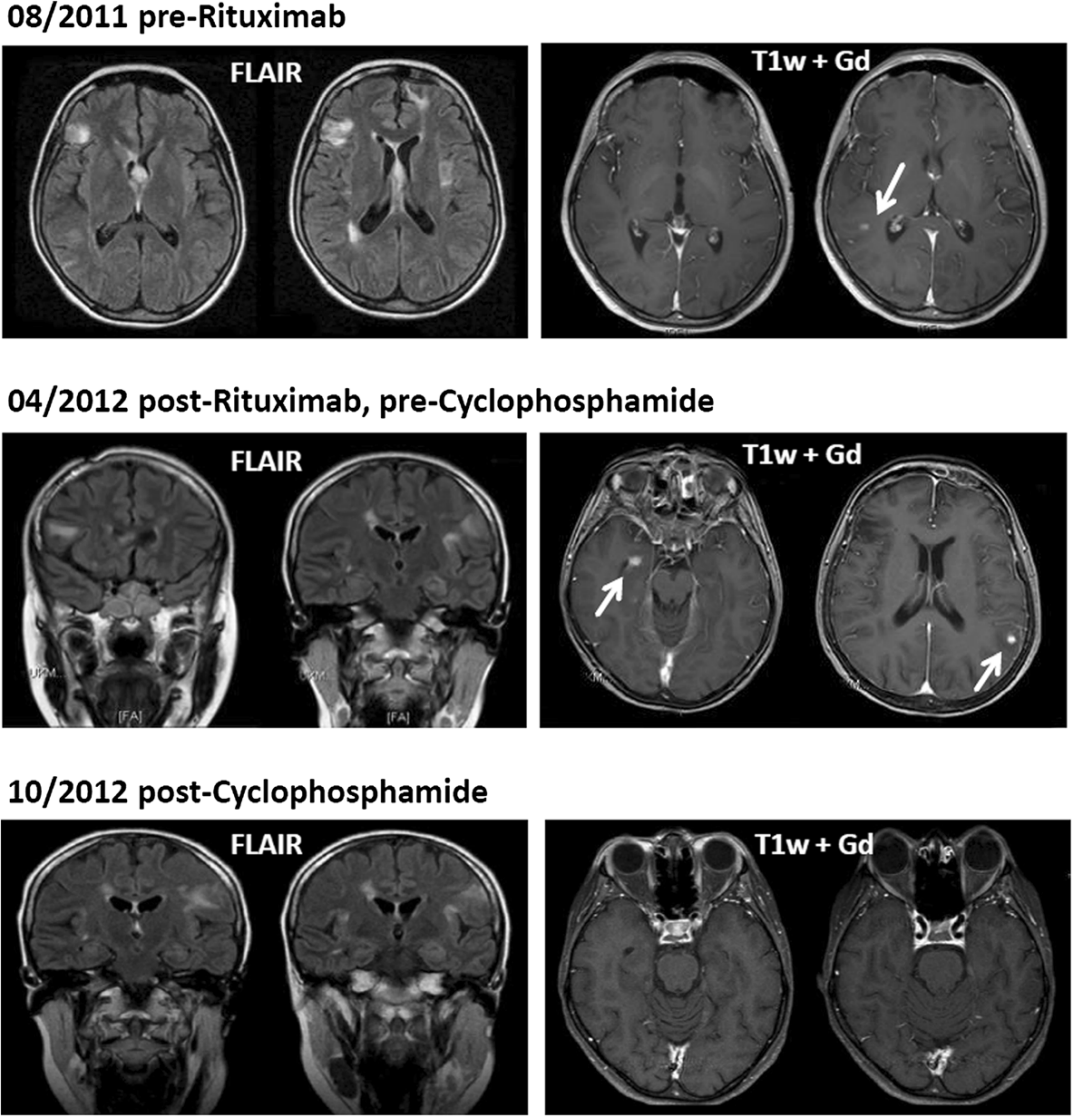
Magnetic resonance imaging time course of the patient during different stages of treatment. Fluid-attenuated inversion recovery and T1-weighted with gadolinium (exemplary images are shown). Arrows indicate gadolinium-enhancing lesions. Treatment with cyclophosphamide resulted in stable disease without new contrast enhancing lesions. FLAIR, fluid-attenuated inversion recovery; Gd, gadolinium.
